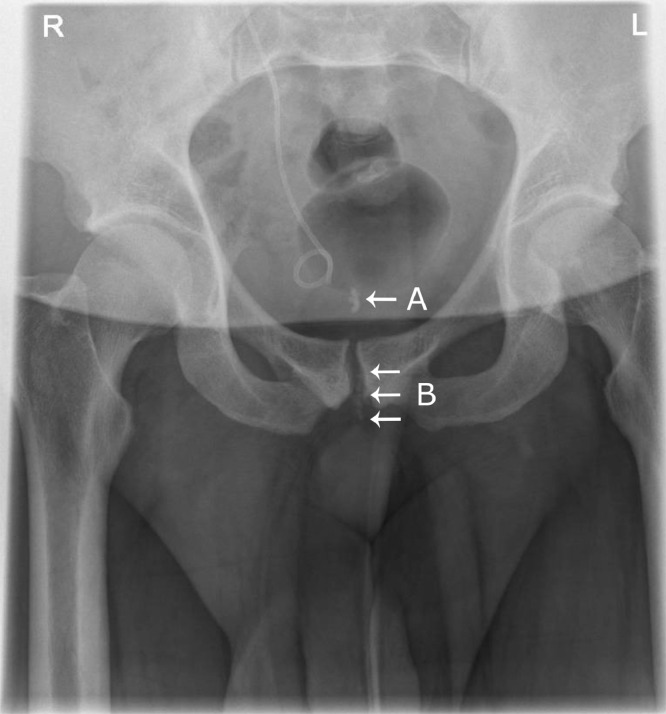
X-Ray of kidneys, ureter and bladder. Anteroposterior radiograph of kidneys, ureter and bladder demonstrates prominent calcifications in the bladder beck (A) and overlying the pubic symphysis (B), capturing a rare string of beads appearance of fragmented stones within the urethra.
